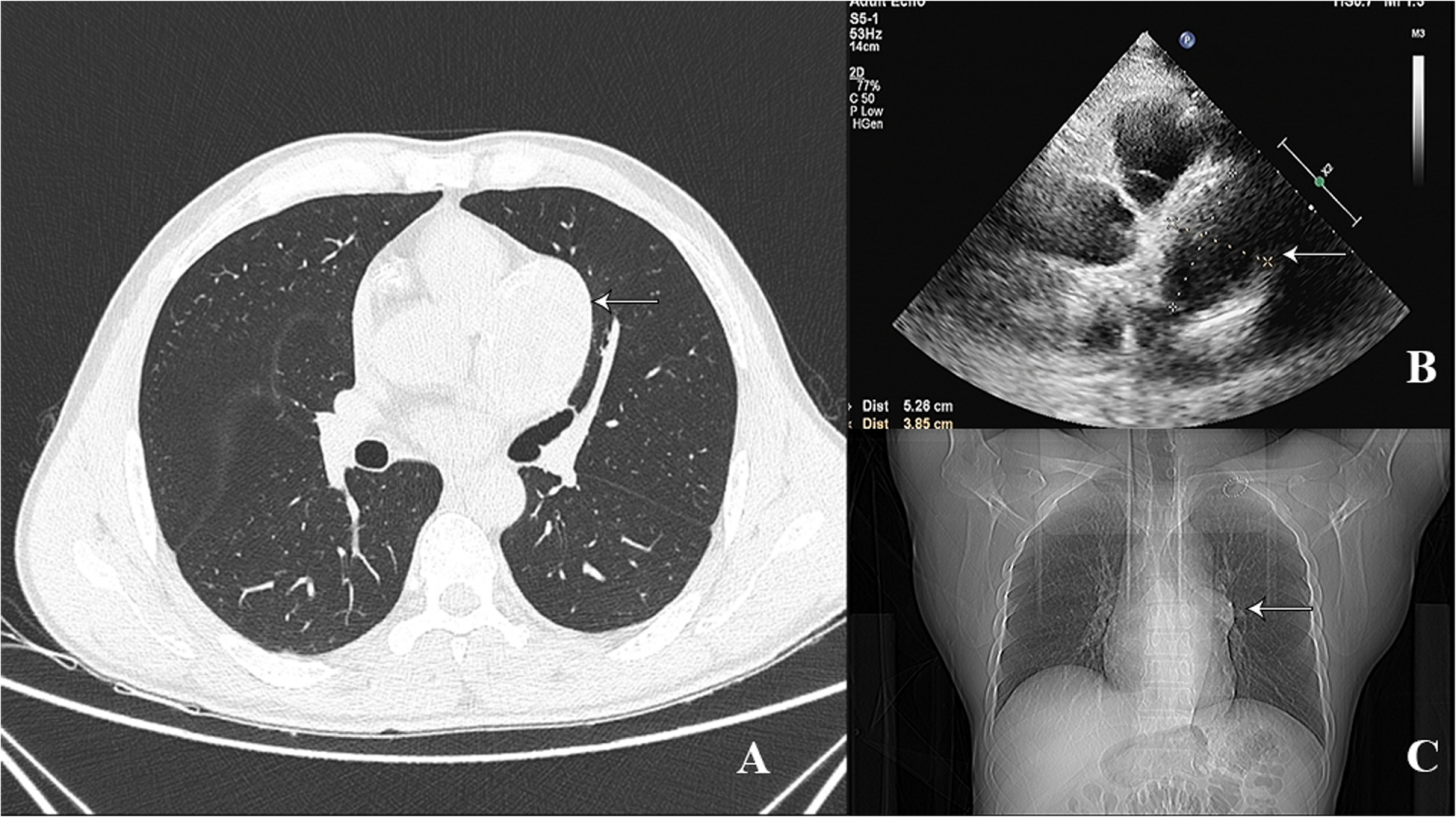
Computed tomography, echocardiography and X-ray examination revealing the aneurysm (arrows)
